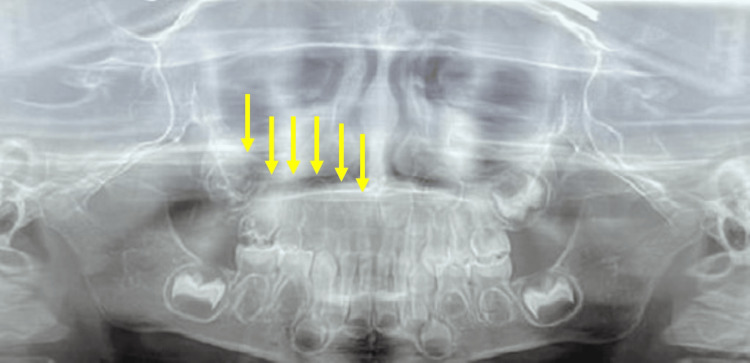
Panoramic radiograph showing the involvement of the upper right primary teeth, with reduced radiopacity, poorly defined crown contours, enlarged pulp chambers, and short or absent roots, consistent with the “ghost tooth” pattern. The germs of the upper right permanent teeth are either absent or show underdevelopment. In contrast, the upper left and lower primary teeth exhibit root and crown development appropriate for the patient’s age
